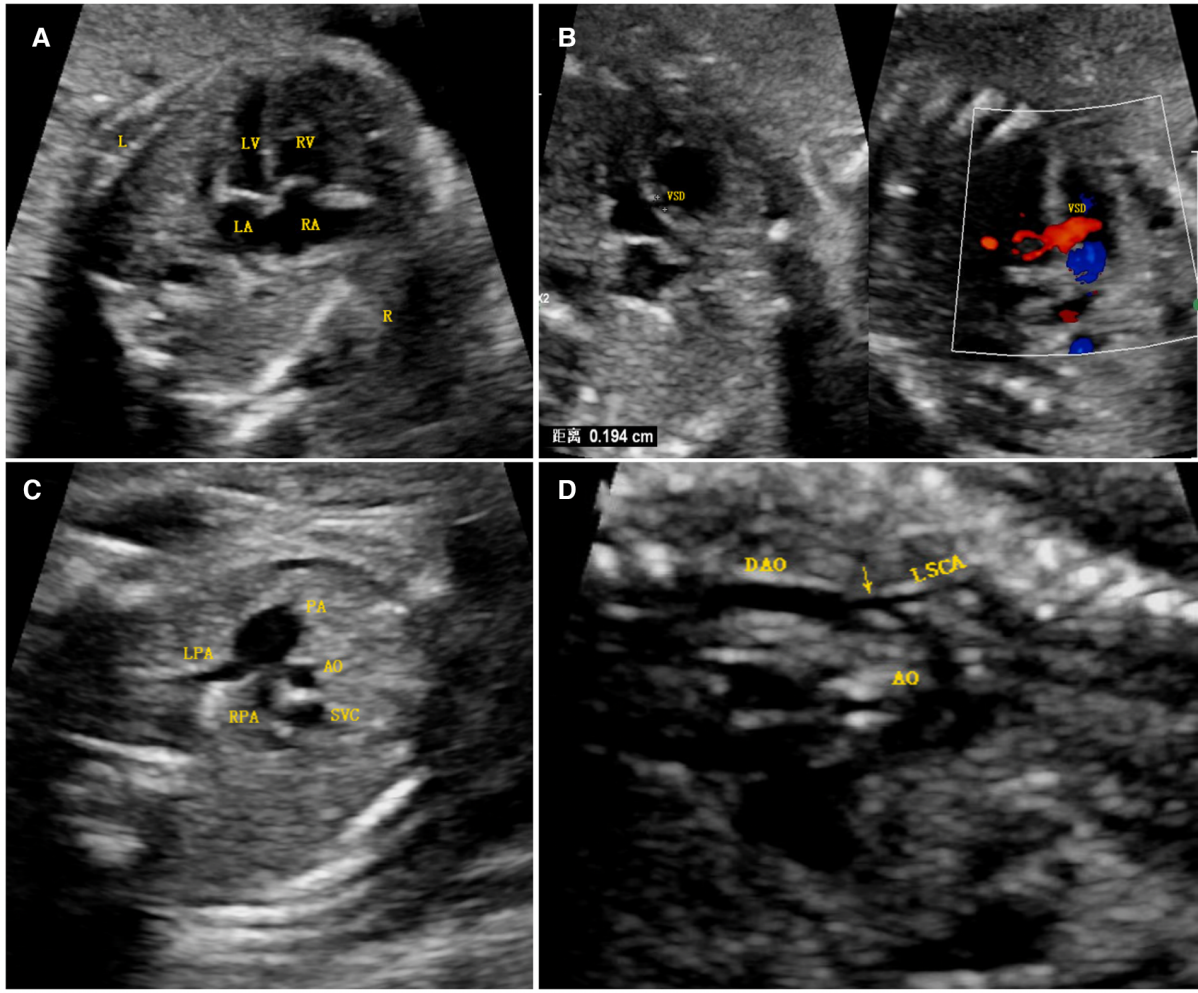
Antenatal ultrasound image. (A) The four-chamber view shows that the right ventricular cavity was significantly larger than the left cardiac cavity, the latter of which was still apex forming. (B) A ventricular septal defect was found just below the aortic outflow, as seen via two-dimensional imaging and color Doppler imaging. (C) A three-vessel view demonstrated a significantly larger main pulmonary artery relative to the aorta (PA > 1.6 AO), the latter of which was smaller than the superior vena cava. (D) A long-axis image of the aortic arch demonstrated diffuse hypoplasia of the arch to the isthmus and a posterior shelf (arrow), in keeping with the aortic coarctation. Abbreviations: LV, left ventricle; RV, right ventricle; LA, left atrium; VSD, ventricular septal defect; RA, right atrium; PA, pulmonary artery; LPA, left pulmonary artery; RPA, right pulmonary artery; SVC, superior vena cava; DAO, descending aorta; LSCA, left subclavian artery; AO, aorta.
